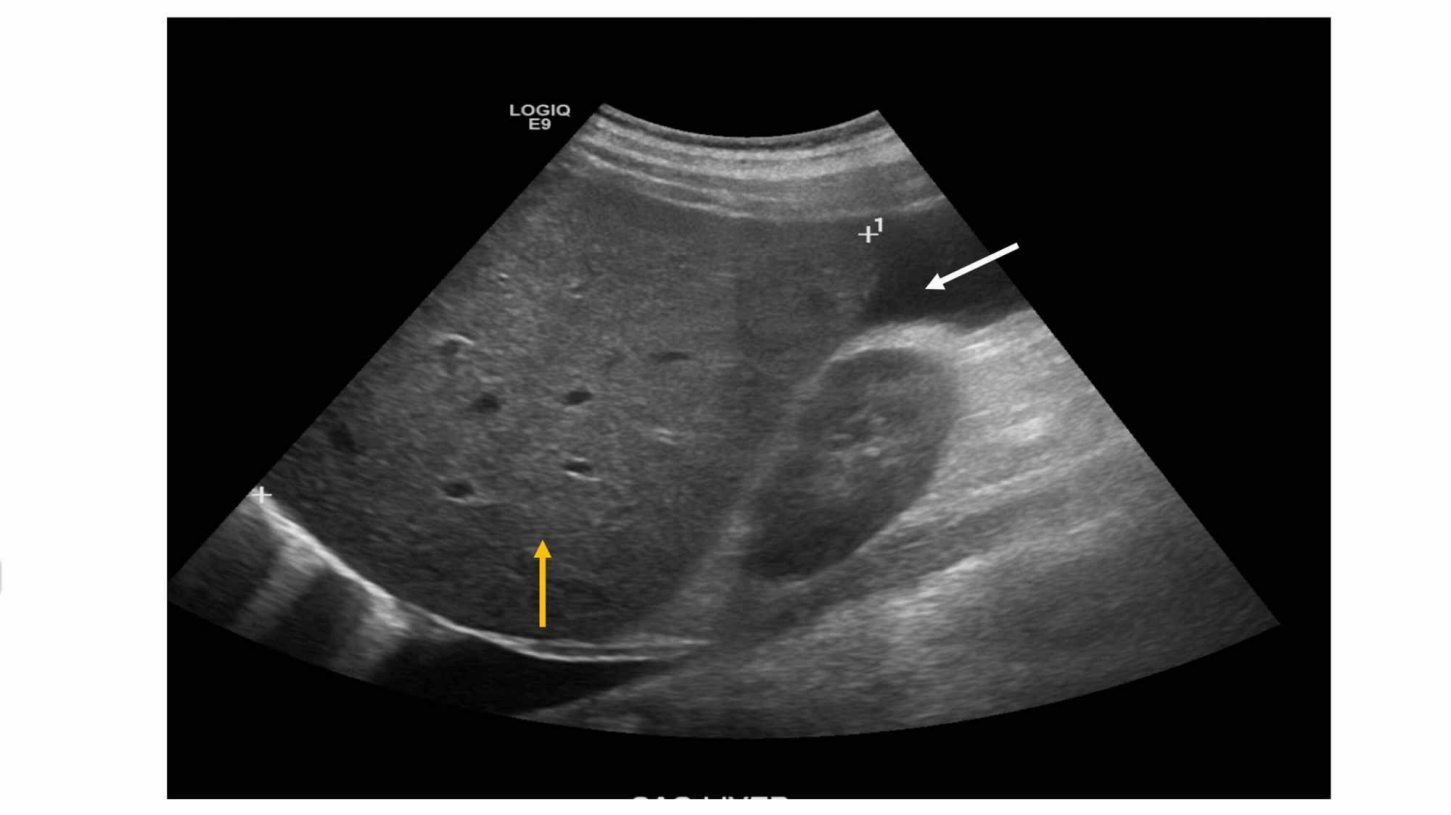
Ultrasound of the abdomen Ultrasound showing hepatomegaly, hepatic steatosis (yellow arrow), and ascites (white arrow).
